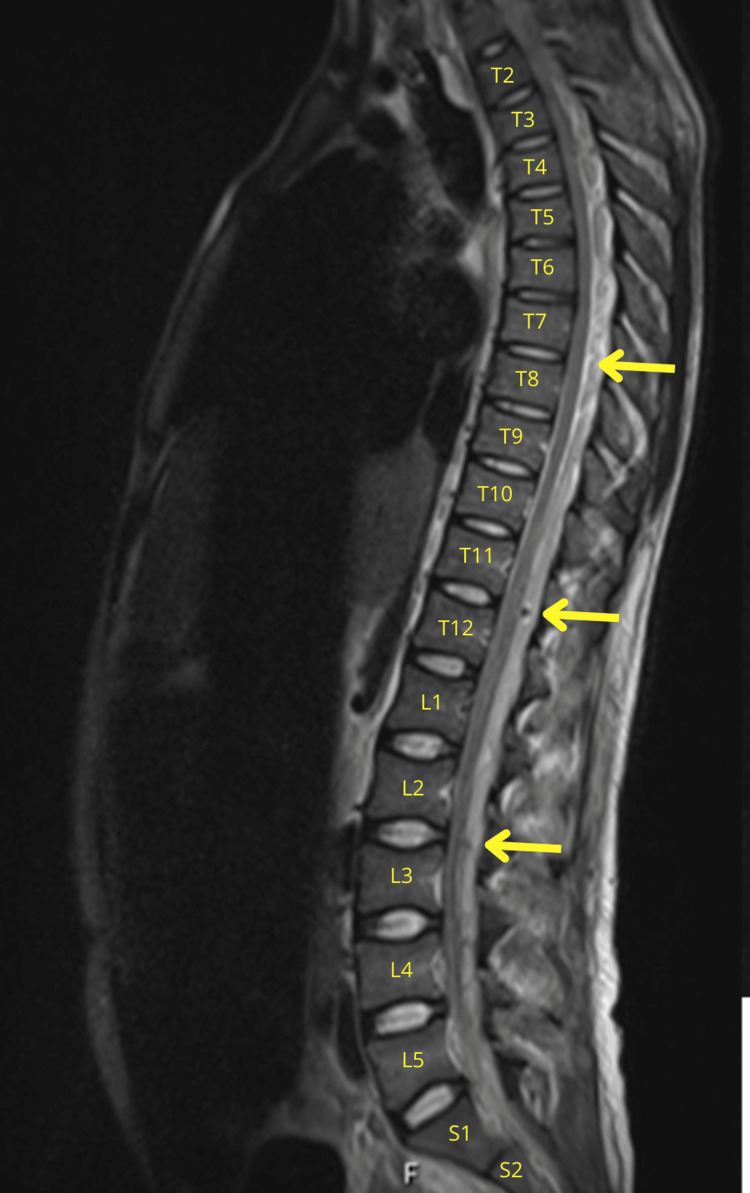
Sagittal thoraco-lumbar MRI scan T2-weighted images reveal an extensive posterior-lateral epidural collection extending continuously up to S2, with maximal thickness ~10 mm at thoracic and lumbar levels (yellow arrows). MRI: magnetic resonance imaging
